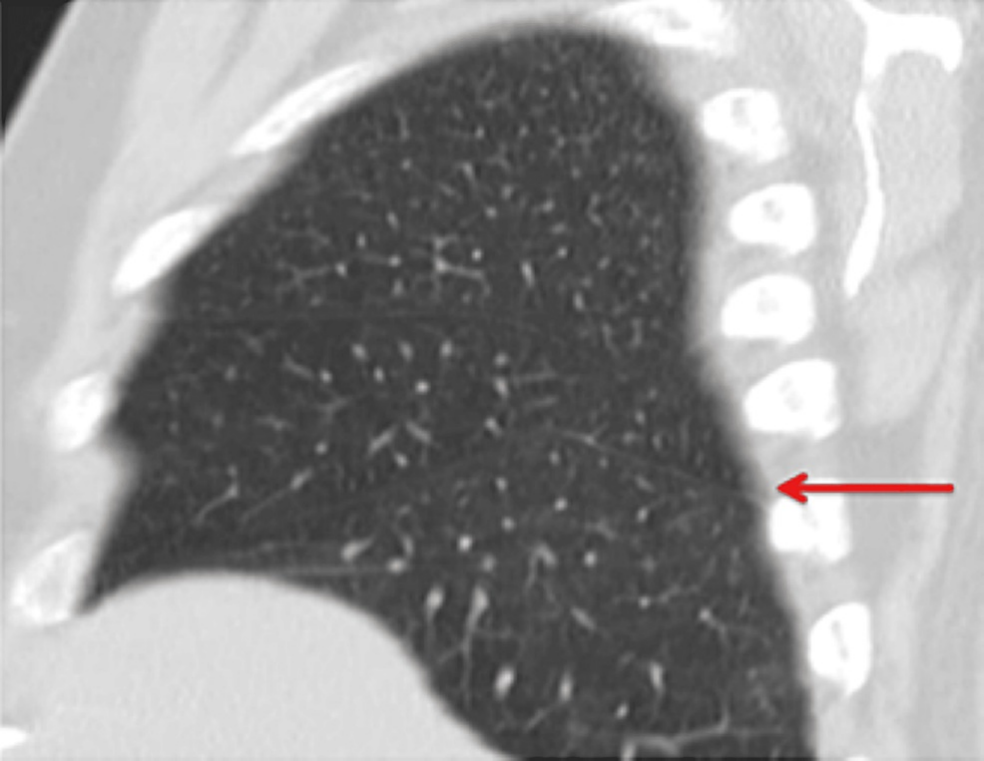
High-resolution computed tomography (HRCT) chest sagittal reformatted image shows thin white line (red arrow) separating the superior segment of the right lower lobe from the remaining segments, the superior accessory fissure
